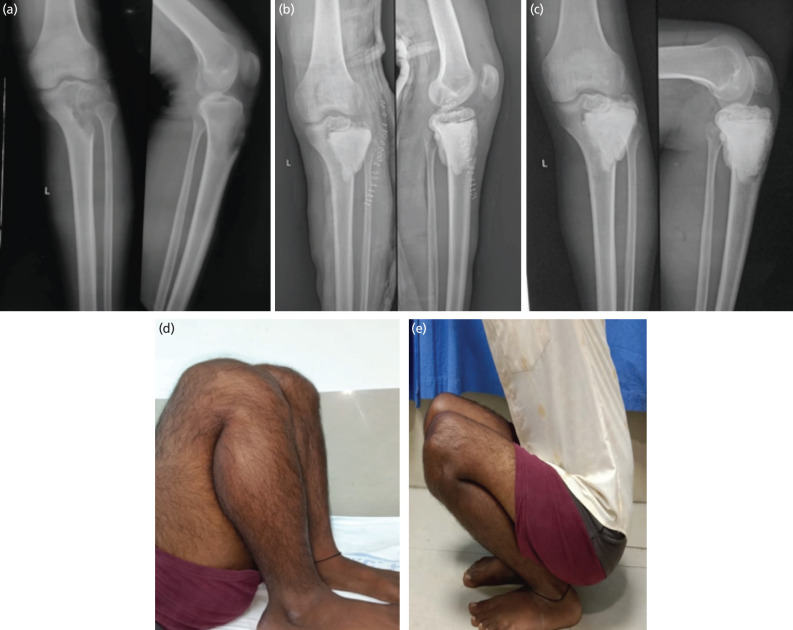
A 20-year-old male with Campanacci grade III GCT of left proximal tibia operated by Sandwich technique. (a) Pre-operative radiograph (AP/Lateral) of left knee with proximal tibia showing lytic lesion extending into intra-articular space with break in cortical continuity, suggestive of Grade III GCT of left proximal tibia. (b) Immediate post-operative radiograph of patient treated with curettage followed by bone grafting and cementation (Sandwich Technique). (c) Radiograph at final two years follow-up showing well healed lesion with no signs of recurrence of GCT. (d and e) Clinical photographs of patient capabilities at two-year follow-up showing full range of motion and complete ability to squat.
